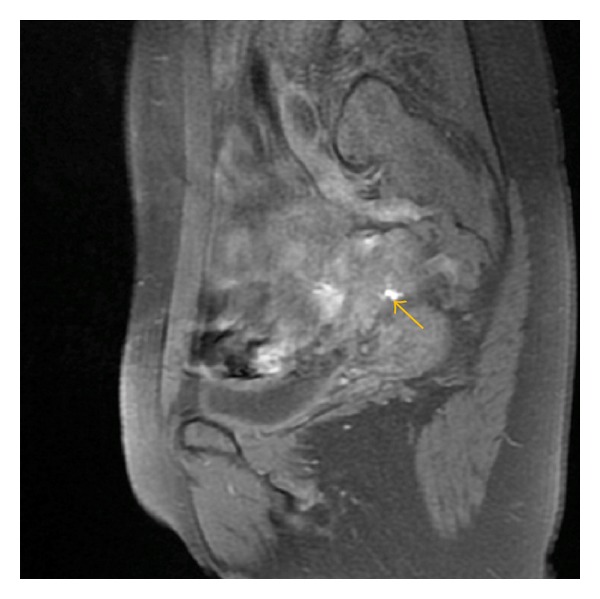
Sagittal T1-weighted fast SE image with fat suppression (TR/TE = 880/7.8) shows small hemorrhagic focus (arrow) in the rectovaginal septum (lesion type II).
